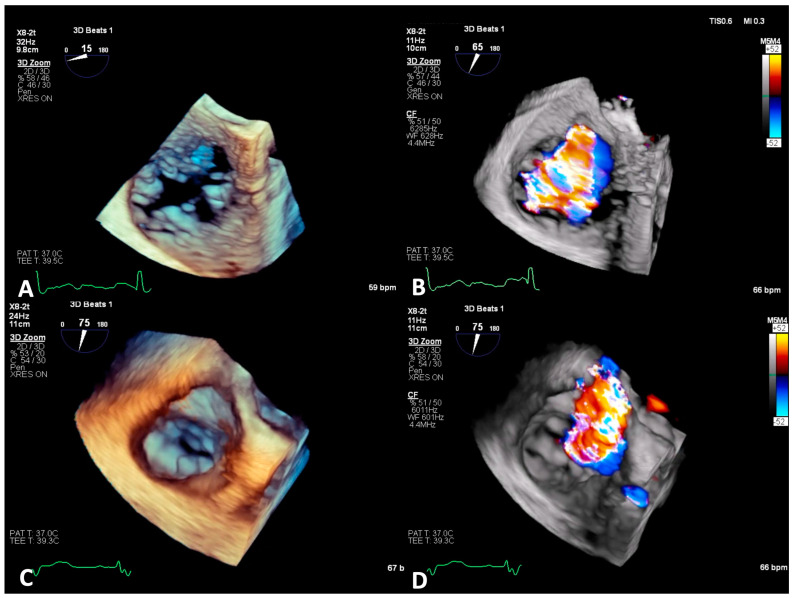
3D zoom without and with color of torrential atrial and ventricular TR. Panels (A,B): 3D zoom TEE of the TV from the mid-esophageal window, in a patient with torrential atrial TR, showing tricuspid annulus dilatation, causing a large coaptation gap, associated with a broad TR jet. Panels (C,D): 3D zoom TEE of the TV from the mid-esophageal window in a patient with ventricular TR due to tethered septal leaflet, resulting in severe TR. TEE, transesophageal echocardiography; TV, tricuspid valve; TR, tricuspid regurgitation.
